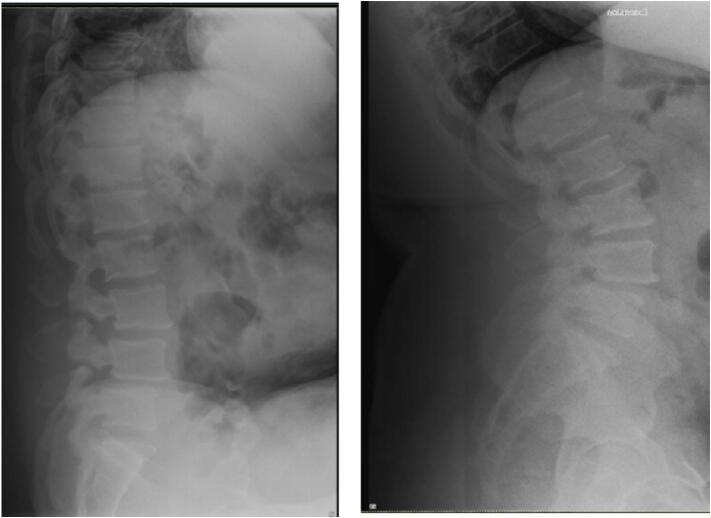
4/10/19 Preoperative Flexion/Extension Lateral Radiographs demonstrating L3–4, L4–5 isthmic spondylolisthesis.
